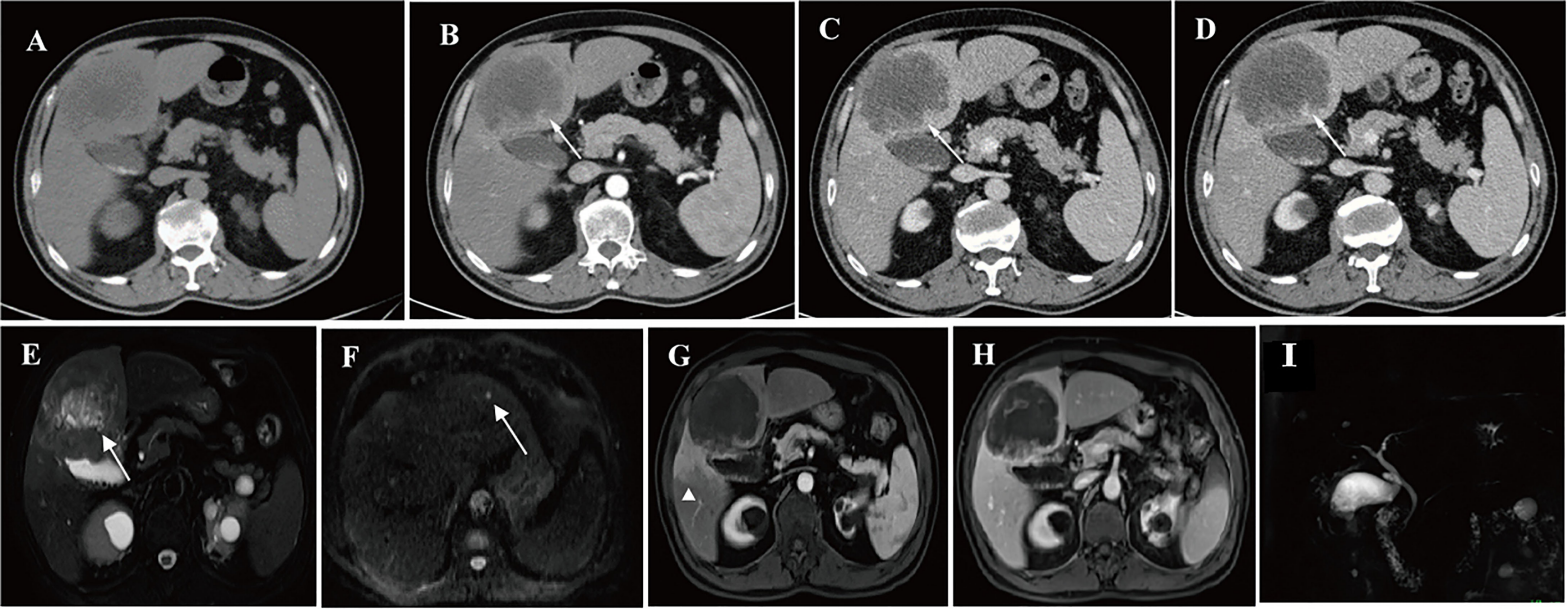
CECT and mpMRI of a representative patient: this 62-year-old man was initially assessed as having a single nodule of intrahepatic cholangiocarcinoma by CECT, while an additional probable nodule was found by MR images with DWI. Pathological examination confirmed that the additional nodule was also ICC, the patients finally received extended liver resection to remove the primary and the secondary tumor module. (A–D) indicate a nodule showing typical features of ICC (rimenhancement in the arterial phase and no washout in the portal venous phase and delayed phase) in dynamic CT images (arrow): (A) pre-contrast phase; (B) arterial phase; (C) portal phase; and (D) delayed phase. (E–I) show dynamic MRI images with DWI and MRCP: (E) shows the same ICC nodule that was detected with DWI (arrow); (F) shows a probable ICC nodule that were found by DWI (arrow); (G) shows peritumor parenchymal arterial phase alteration (arrowhead) and no washout in portal venous phase (H); and no biliary dilation displays in (I).
